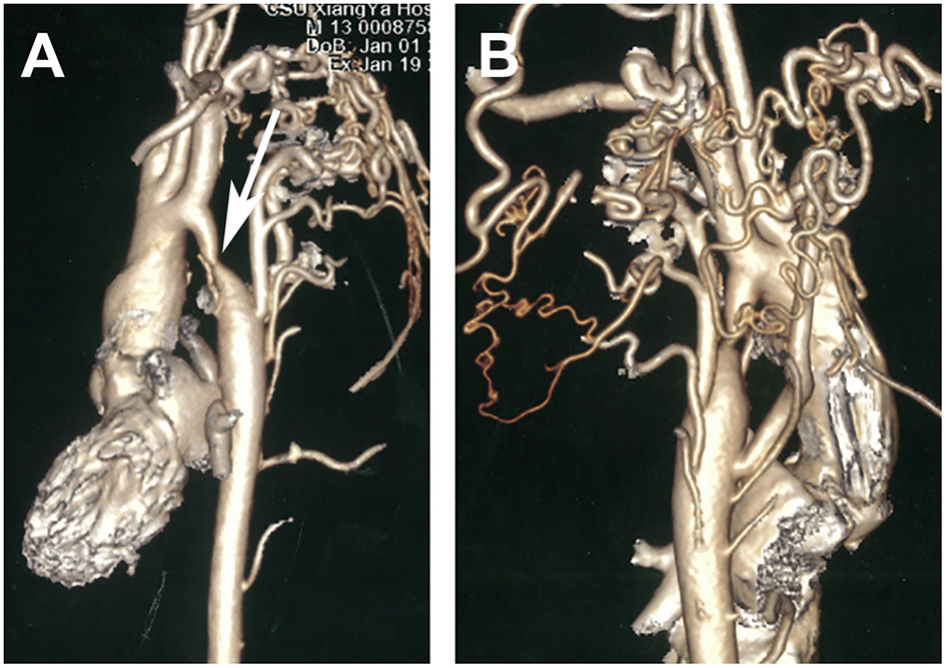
Computed tomography angiography preoperatively showing a coarctate descending aorta [(A), arrow] with more than eight collateral vessels between the proximal and distal coarctated tissue (B).
